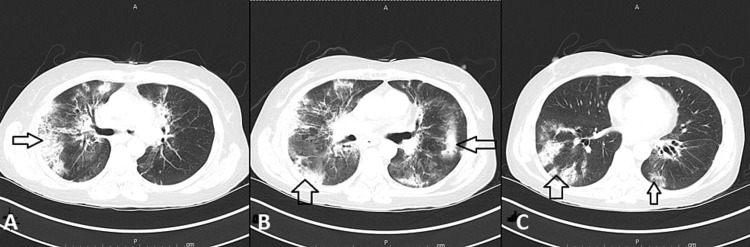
Chest Computed Tomography Even further progression was seen in a repeat CT scan of the chest in December 2022 (Arrows in A, B, and C).
